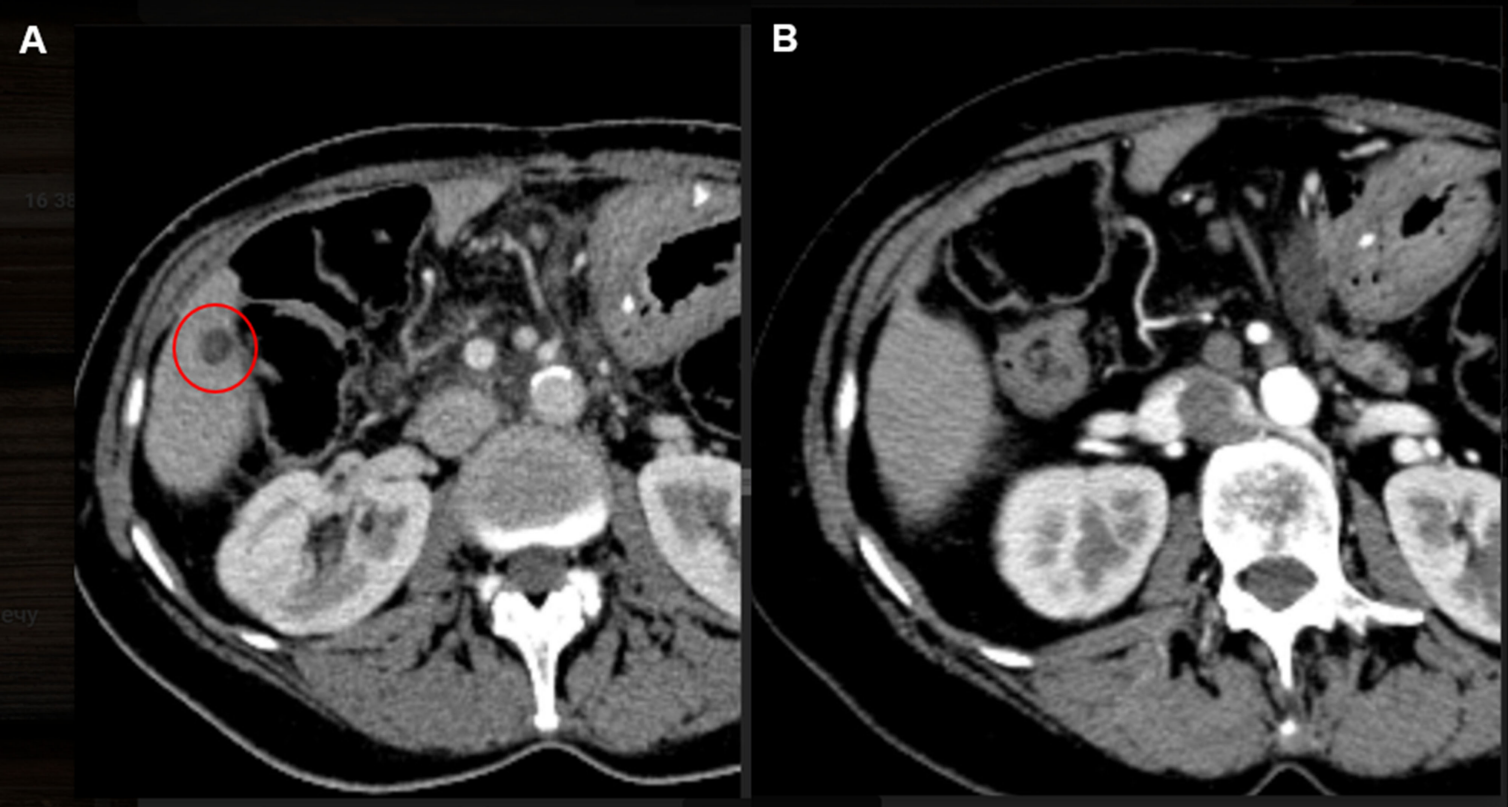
(A) CT scan of S6 liver lesion, 11 mm in greatest dimension before start of talazoparib treatment (March 2019), (B) CT scan of S6 of the liver after 25 months of treatment without visual evidence of the disease (April 2021).
